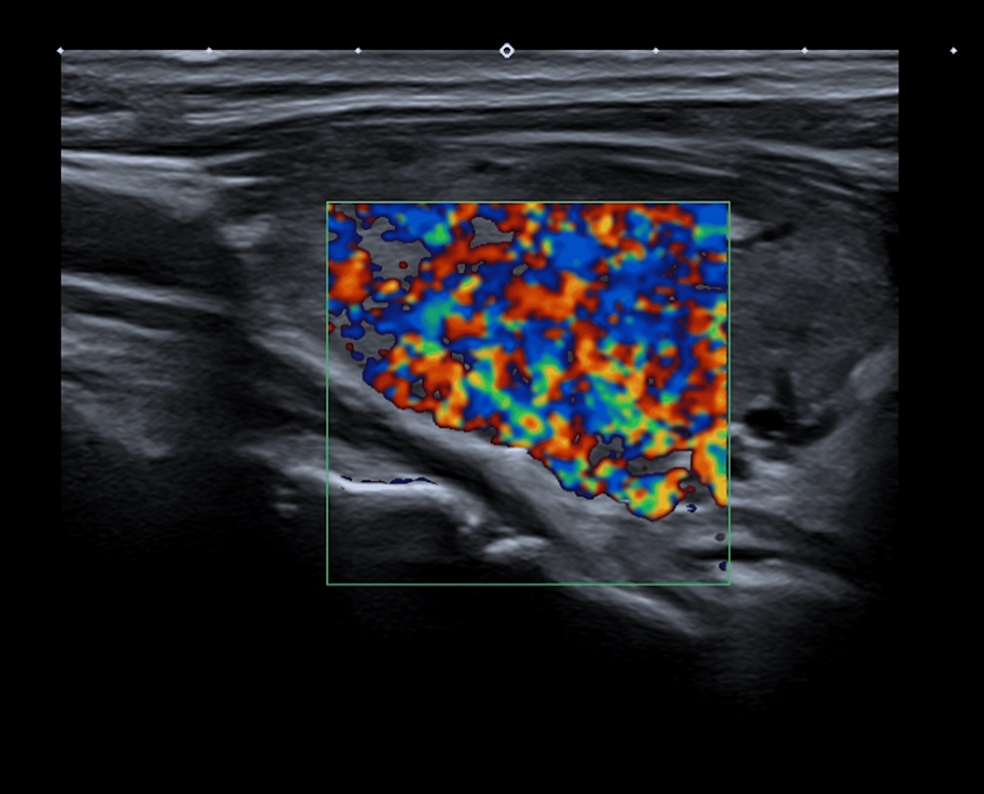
Thyroid ultrasonography with doppler
